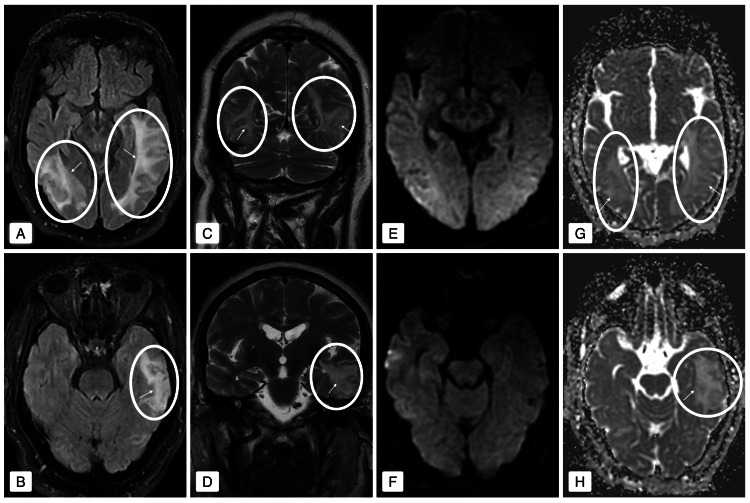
Brain magnetic resonance imaging (MRI). (A-D) Axial FLAIR and coronal T2-weighted images demonstrate subcortical hyperintensities with a digitiform pattern, predominantly affecting the occipital lobes and the left temporal lobe. (E-H) Diffusion-weighted imaging (DWI) shows no signal intensity abnormalities; however, the apparent diffusion coefficient (ADC) maps reveal elevated values, confirming the presence of vasogenic edema (circles and arrows highlight the areas of lesion).
